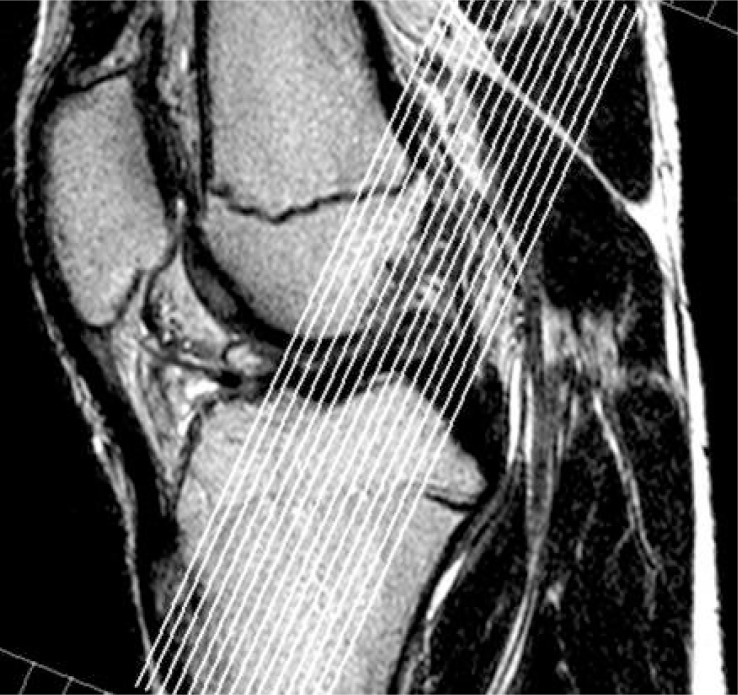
Sagittal image of standard MRI examination as a topogram for planning the paracoronal oblique T2 FSE 2 mm image.
